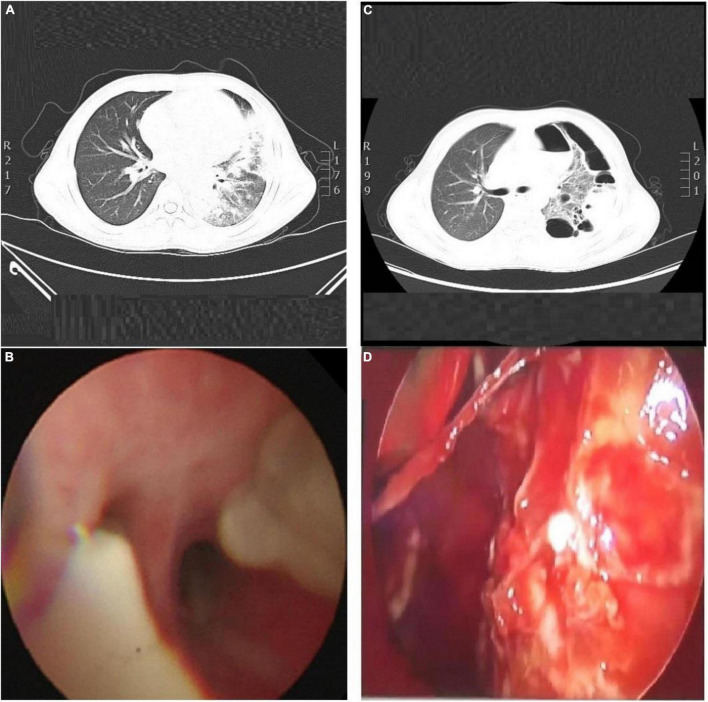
(A) CT scan of his chest, show the left lung and the right lower lobe consolidation, with atelectasis in the lower lobe of the left lung. The left pleural cavity showed a small amount of pleural effusion, and the lumen of the bronchial branch of the left lower lobe was not unobstructed. (B) Bronchoscopy, show the basal segment of left lower lobe, the bronchial mucosa was rough, a large number of yellow and white mucus plugs were found in the opening, and the ventilation was not smooth. (C) Chest CT scan. Indicate consolidation of left and right upper and lower lobes with signs of atelectasis and multiple cavities in the left lung, partially wrapped left pneumothorax. (D) Thoracoscopy, show the lung surface covered with a yellow purulent moss-like layer.
